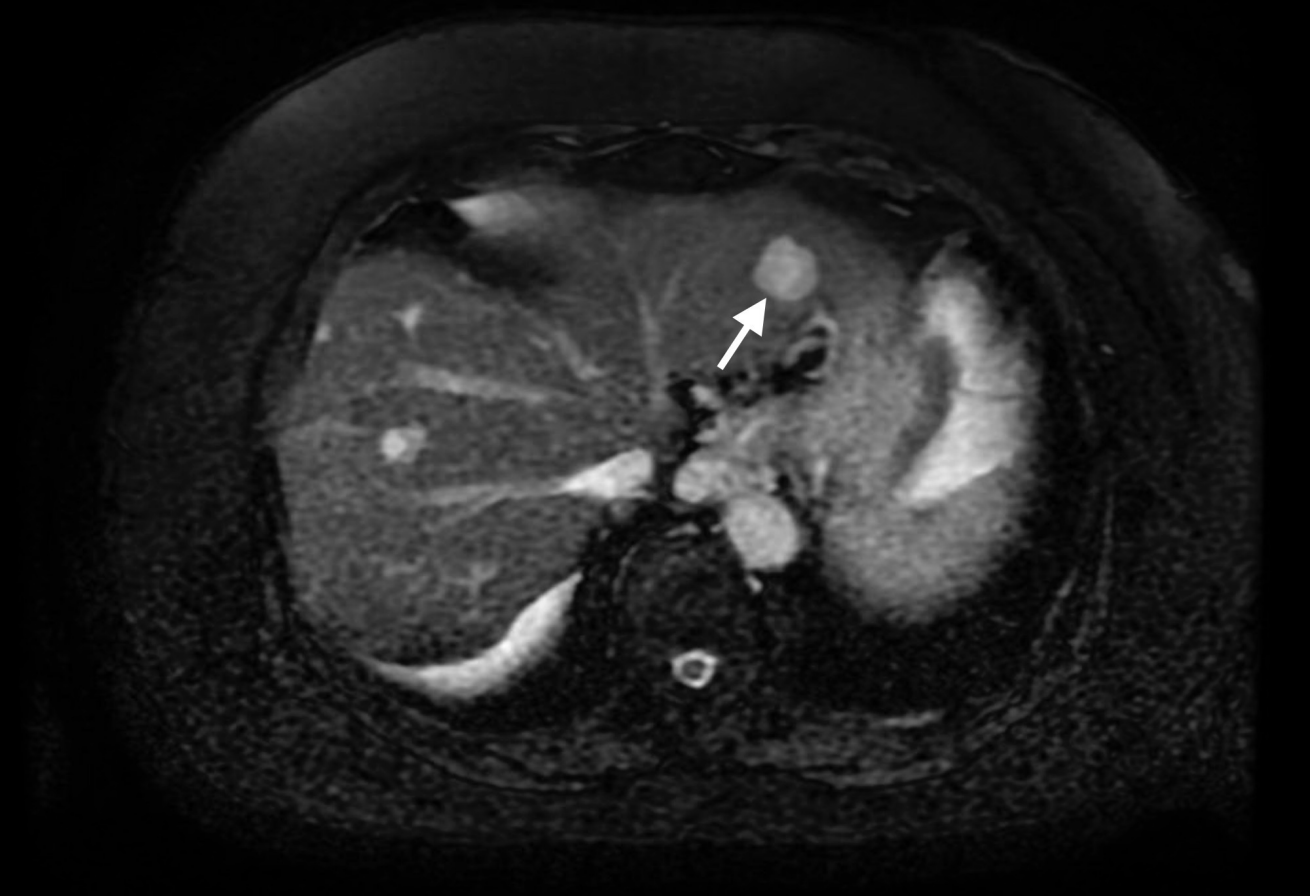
Abdominal computed tomography scan demonstrating 2-cm nodular mass in the left lobe of the liver
